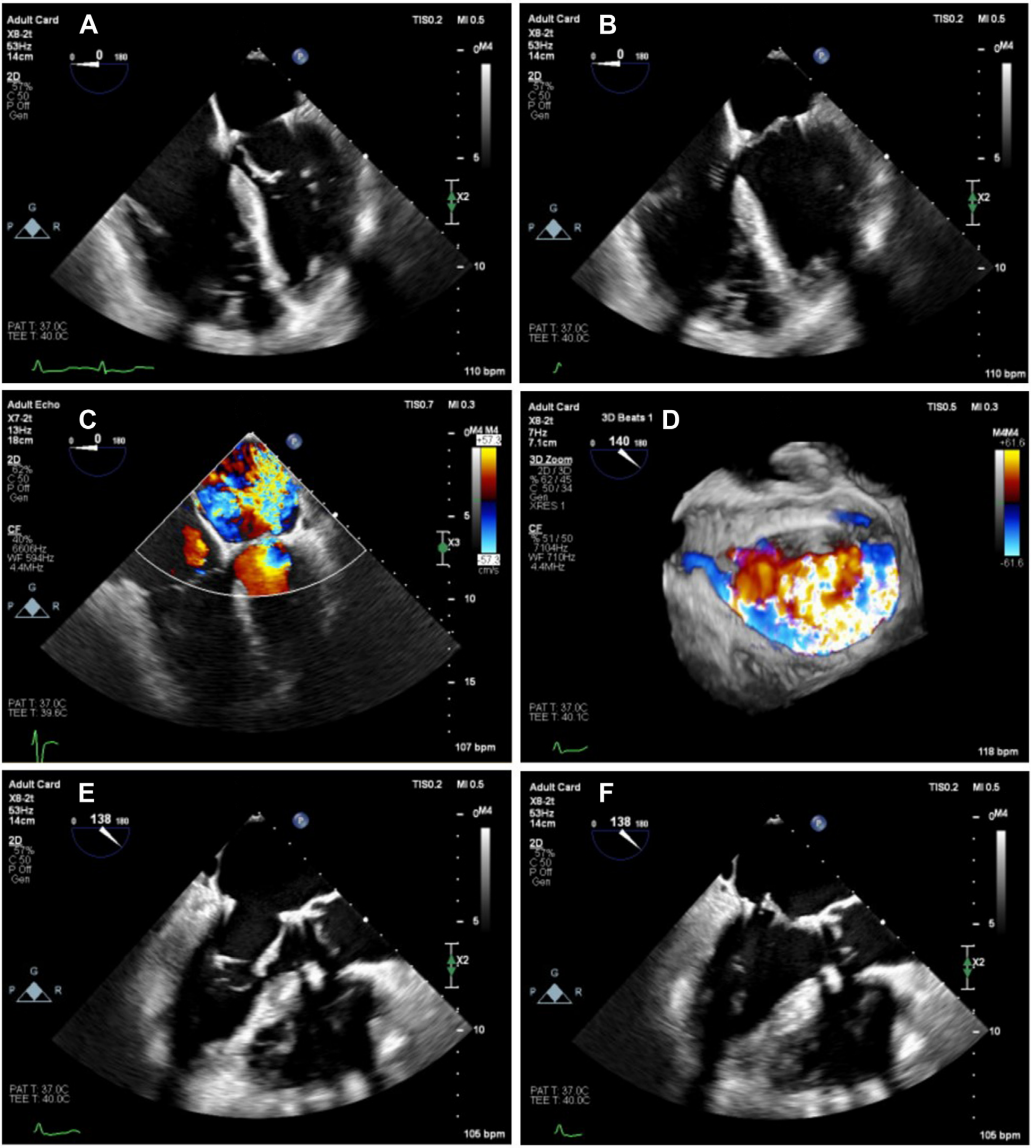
Transesophageal Echocardiography Opening (A and E) and closing (B and F) of the mitral valve in the 4-chamber and left ventricular outflow tract views, respectively. (C and D) The posteriorly directed jet of severe mitral regurgitation in the 4-chamber and 3-dimensional en-face views, respectively.
